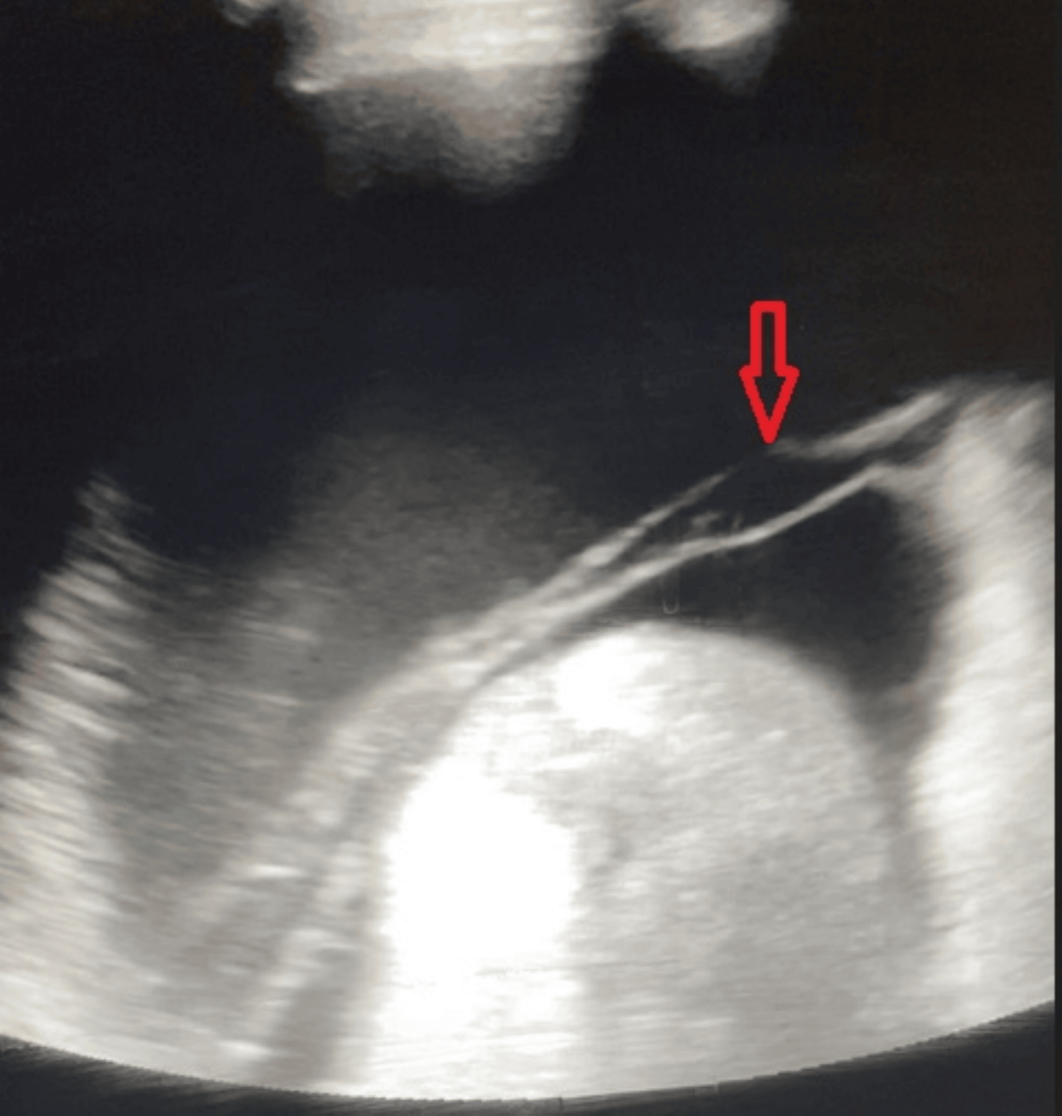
Initial ultrasound findings before the first paracentesis. Findings were consistent with mild loculated ascites and fibrinous strands forming multiple loculations (red arrow).
